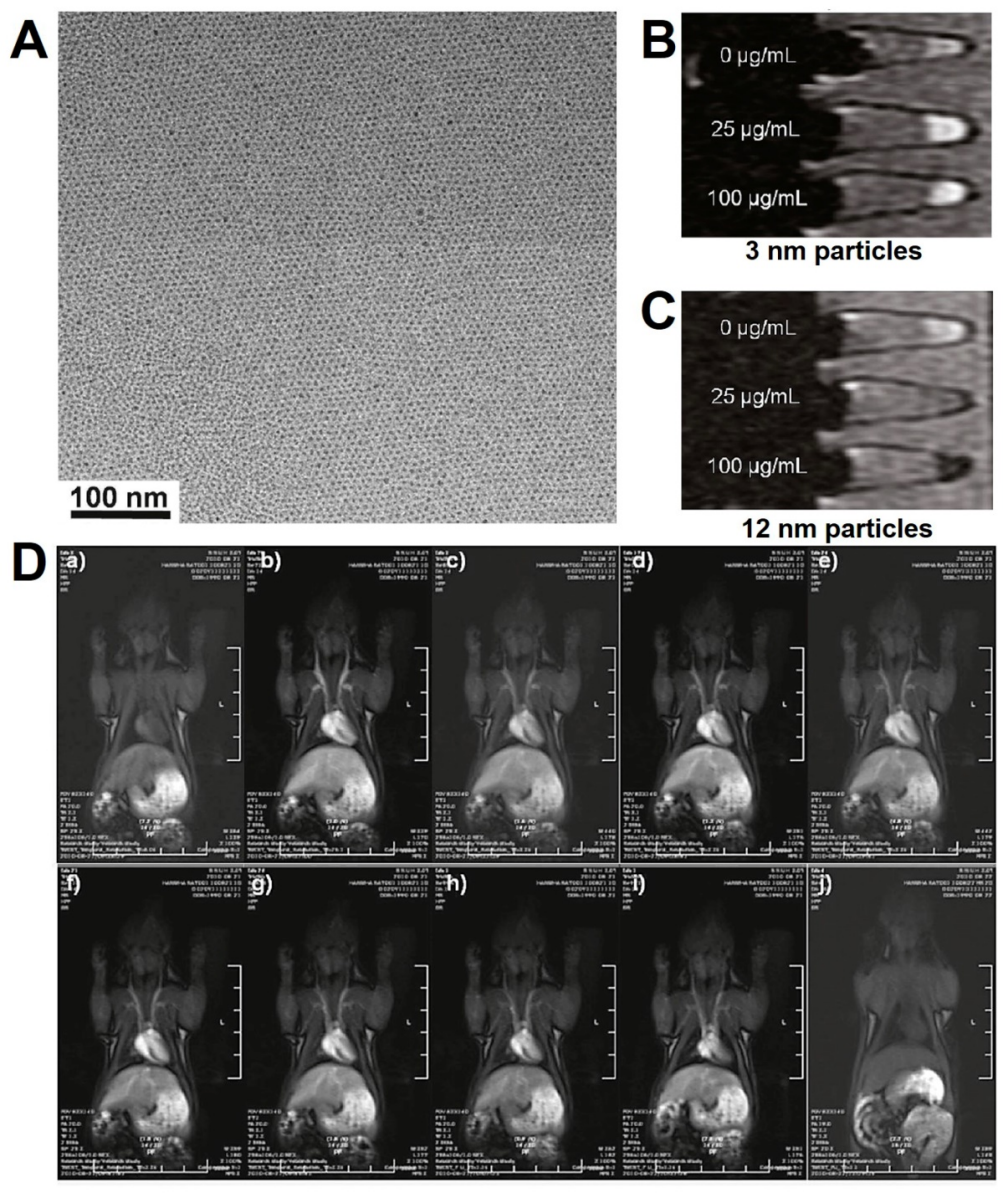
(A) TEM images of Fe_3_O_4_ NPs; *T*_1_-weighted MRI of Fe_3_O_4_ NPs with the diameters of 3 nm (B) and 12 nm (C); (D) The MRI intensity with the dynamic time *in vivo.* Adapted with permission from 189, copyright 2011 Journal of the American Chemical Society.
